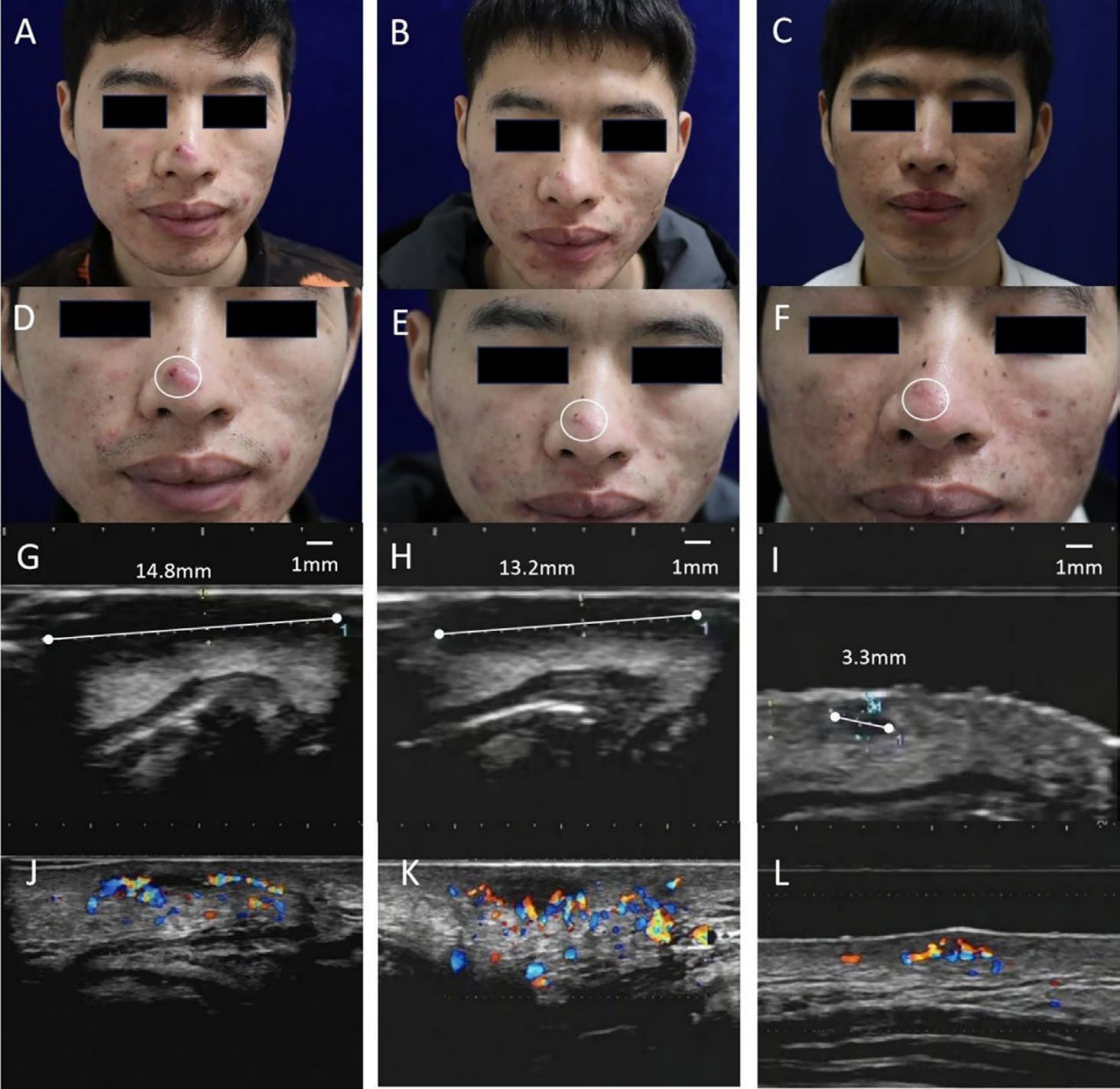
Clinical, ultrasonographic, and Doppler findings in a 20‐year‐old male with moderate‐to‐severe acne vulgaris. Clinical progression: (A) Baseline: Facial presentation showing inflammatory papules, pustules, cysts, and scarring. (B) Week 4: Reduced papule and pustule count with persistent cysts. (C) Week 12: Significant clinical improvement; minimal residual erythematous papules and scarring. Severest lesion evolution & Pillsbury grading: (D) Baseline: Large cyst (white circle); Pillsbury grade: Severe. (E) Week 4: Most severe lesion: Inflammatory papule (white circle); Pillsbury grade: Moderate. (F) Week 12: Most severe lesion: Scar (white circle); Pillsbury grade: Mild. HFUS (18 MHz) findings: (G) Baseline: Dermal‐subcutaneous pseudocyst (14.8 × [width] mm; > 5 mm depth), extending deep to adipose layer with indistinct borders and posterior acoustic enhancement (SSSA Grade III). (H) Week 4: Persistent pseudocyst (13.2 × [width] mm; SSSA Grade III). (I) Week 12: Significant resolution; residual focal dermal hypoechoic focus (3.3 × [width] mm; SSSA Grade II). CDFI: (J) Baseline: Marked intralesional vascularity. (K) Week 4: Persistent abundant vascularity. (L) Week 12: Minimal residual vascular flow.
